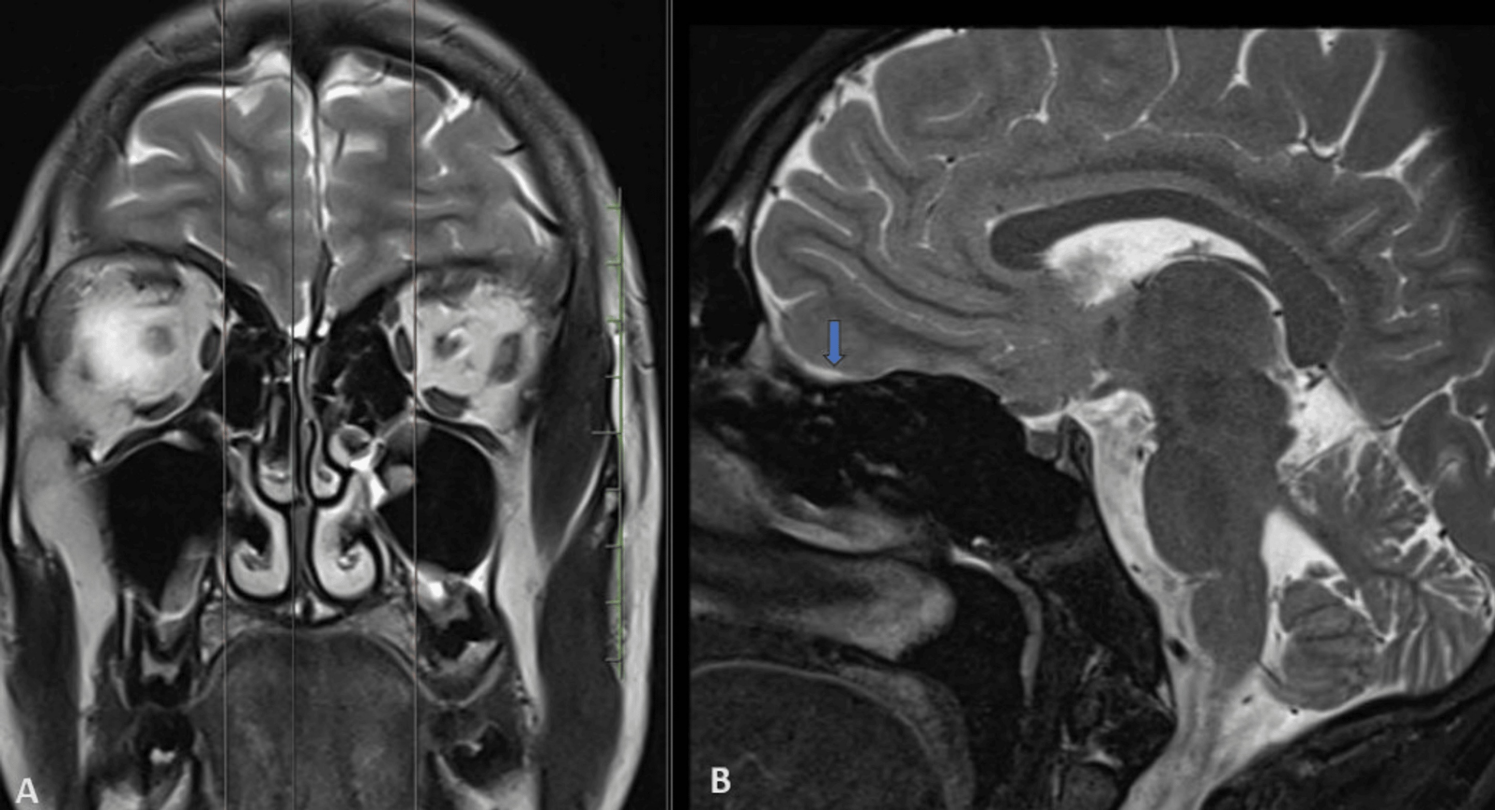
T2-weighted MRI in coronal section (A) with sagittal section (B) at corresponding level show absent olfactory bulb in right olfactory groove with hypoplastic olfactory sulcus (blue arrow).
